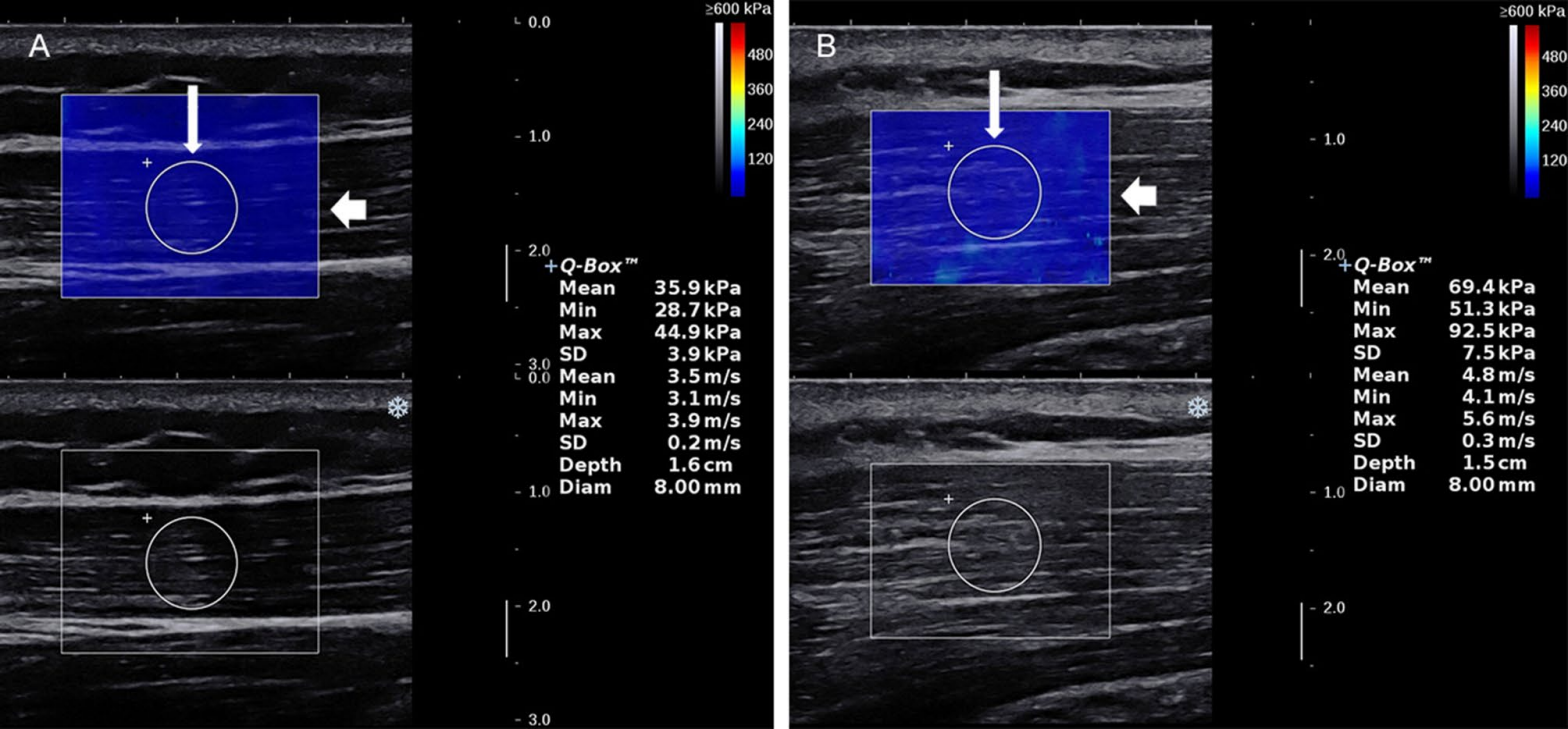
SWE-mode (top panel) and B-mode (bottom panel) ultrasound images of the UT. A color map of UT elasticity is shown in the square region of interest (ROI) (thick arrow). The mean SWV in the round Q-box (thin arrow) is presented on the right side of the image. A,B show that the size of the Q-box is 8 mm in diameter. The mean SWV of a healthy volunteer at R0° was 3.5 m/s (A), the mean SWV at R90° was 4.8 m/s (B).
